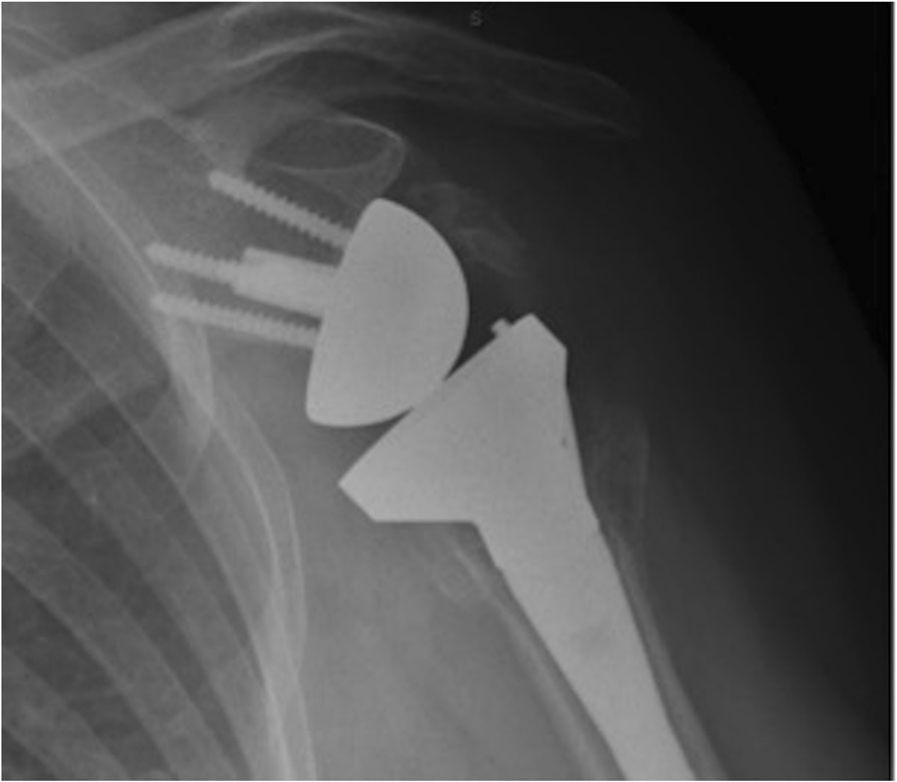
Anteroposterior radiographs of BIO-RSA with Sirveaux grade 2 scapular notching
